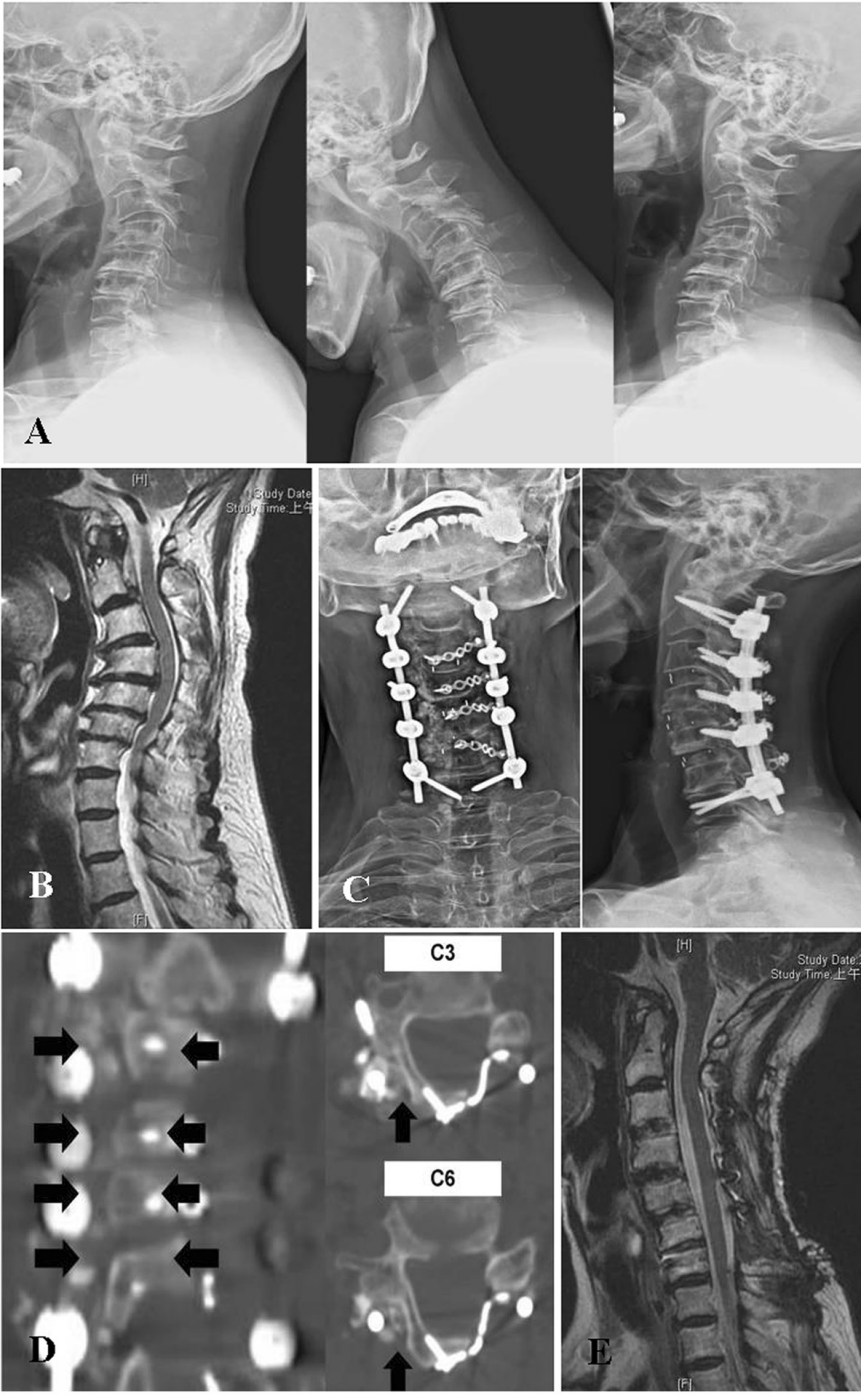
A 55-year-old woman underwent C3-6 anterior diskectomy with fusion, posterior instrumented fusion with C2, C7 pedicle screws and C3-5 lateral mass screws, and posterior decompression with C3-6 laminoplasty. a Preoperative X-rays show fixed cervical kyphosis with C2-7 marked spondylosis. b Preoperative midsagittal T2-weighted magnetic resonance imaging shows that C2-7 stenosis with spinal cord compression. c Postoperative X-rays show that cervical curvature has been restored and the fusion structure is solid. d Postoperative 6 months computed tomography scans demonstrate that hinge side fusion bed union (*black arrows*). e Postoperative 12 months midsagittal T2-weighted magnetic resonance imaging shows patent spinal cord without compression
